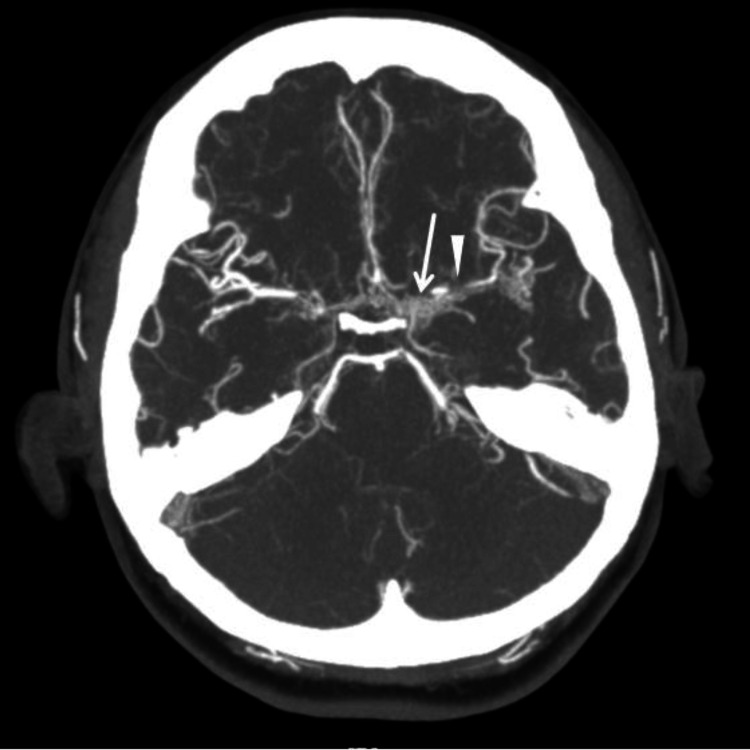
CT angiography of the brain shows a small patent left ICA which becomes subtotally occluded at the carotid siphon (arrowhead) with multiple collaterals around the proximal MCA vessels (arrow). The findings are suggestive of a moyamoya pattern or less likely an unusual vasculitis. ICA: internal carotid artery; MCA: middle cerebral artery
